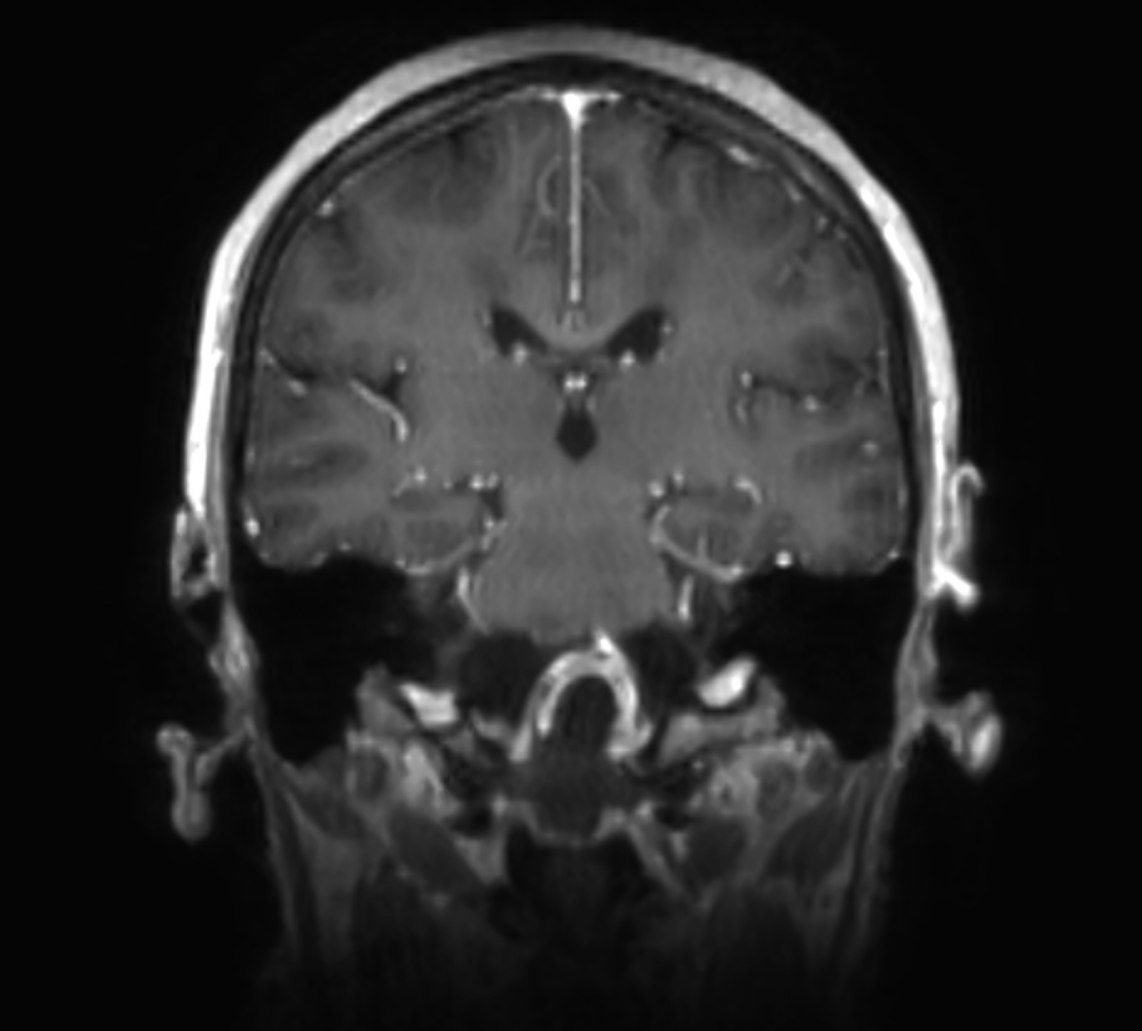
September Coronal MRI Enhancing mass
